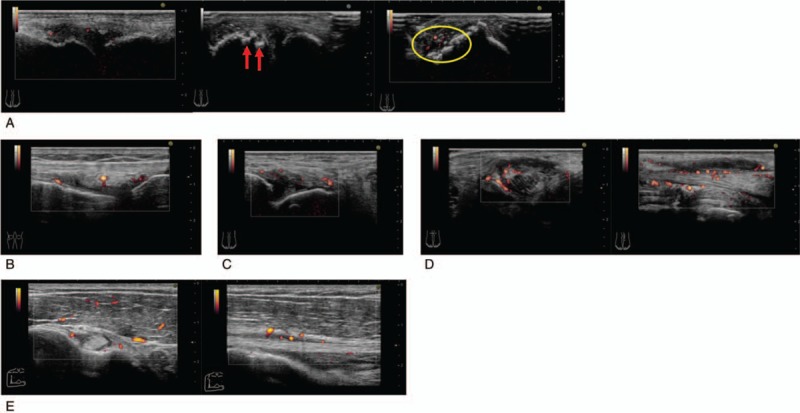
Musculoskeletal ultrasound (MSUS) at the first visit revealed active synovitis. (A) First metatarsophalangeal (MTP) joints of both feet had bone erosion (red arrows), crystal aggregates (yellow circle), and synovial thickening with power Doppler signals. (B) Right knee joint and (C) left ankle joint also had synovial thickening with power Doppler signals. (D) Left posterior tibial tendon and (E) right bicep tendon showed tendon sheath thickening with power Doppler signals.
